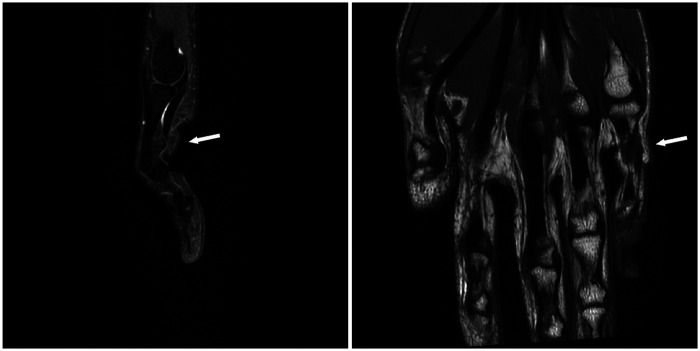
Preoperative MRI examination: sagittal and coronal images of the patient's left little finger.
